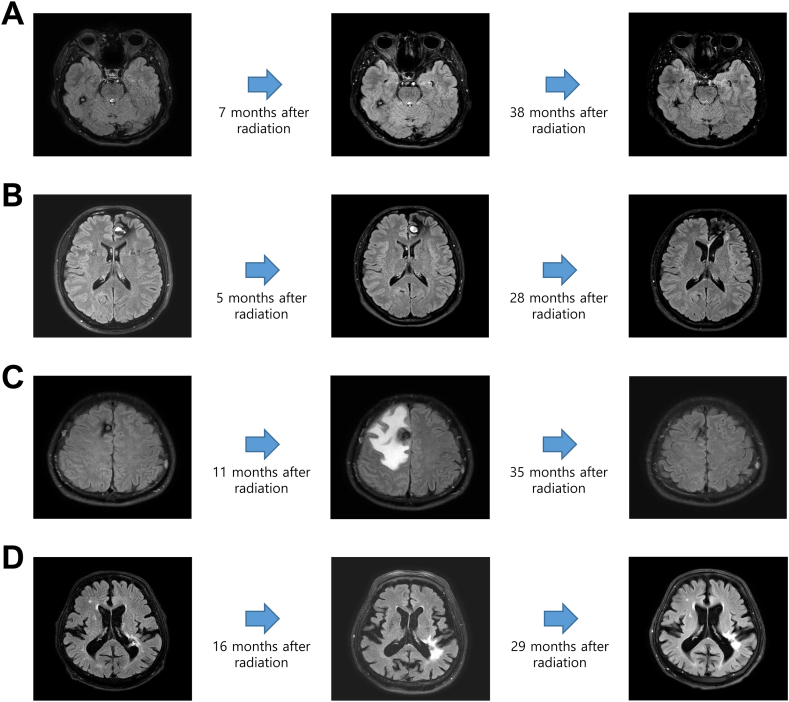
(A) A 31-year-old male patient suddenly developed blurred vision. MRI revealed a CCM in the right temporal area and SRS treatment with 18 Gy was administered. Nidus volume reduction was observed 38 months after SRS treatment; (B) A 39-year-old male patient presenting with repeated seizure attacks. MRI revealed a cystic CCM in the left frontal lobe and SRS treatment with 18 Gy was administered. Nidus volume reduction was observed 28 months after SRS treatment; (C) A 45-year-old male patient presenting with persistent headache and dizziness. MRI revealed a CCM in the right high frontal lobe and SRS treatment with 18 Gy was administered. Eleven months after radiation treatment, the patient presented with left hemiparesis and was admitted. MRI revealed an intranidal hemorrhage with severe PBE. The patient was treated with steroids for 4 months to control the brain edema. Thirty-five months after SRS treatment, the patient recovered completely and the last follow-up MRI showed CCM nidus volume decrease and no PBE. (D) An 81-year-old male patient presenting hemiparesis due to cerebral hemorrhage. MRI revealed a CCM located in the left posterior paraventricular area and SRS treatment with 17 Gy was administered. Sixteen months after SRS treatment, follow-up MRI showed nidus volume decrease with asymptomatic PBE. The patient was treated with steroid for 3 months. Twenty-nine months after radiation treatment, the patient's symptoms improved and the last follow-up MRI showed CCM nidus volume decrease and persistent but slightly decreased asymptomatic PBE. CCM, cerebral cavernous malformation; MRI, magnetic resonance imaging; PBE, perilesional brain edema; SRS, stereotactic radiosurgery.
